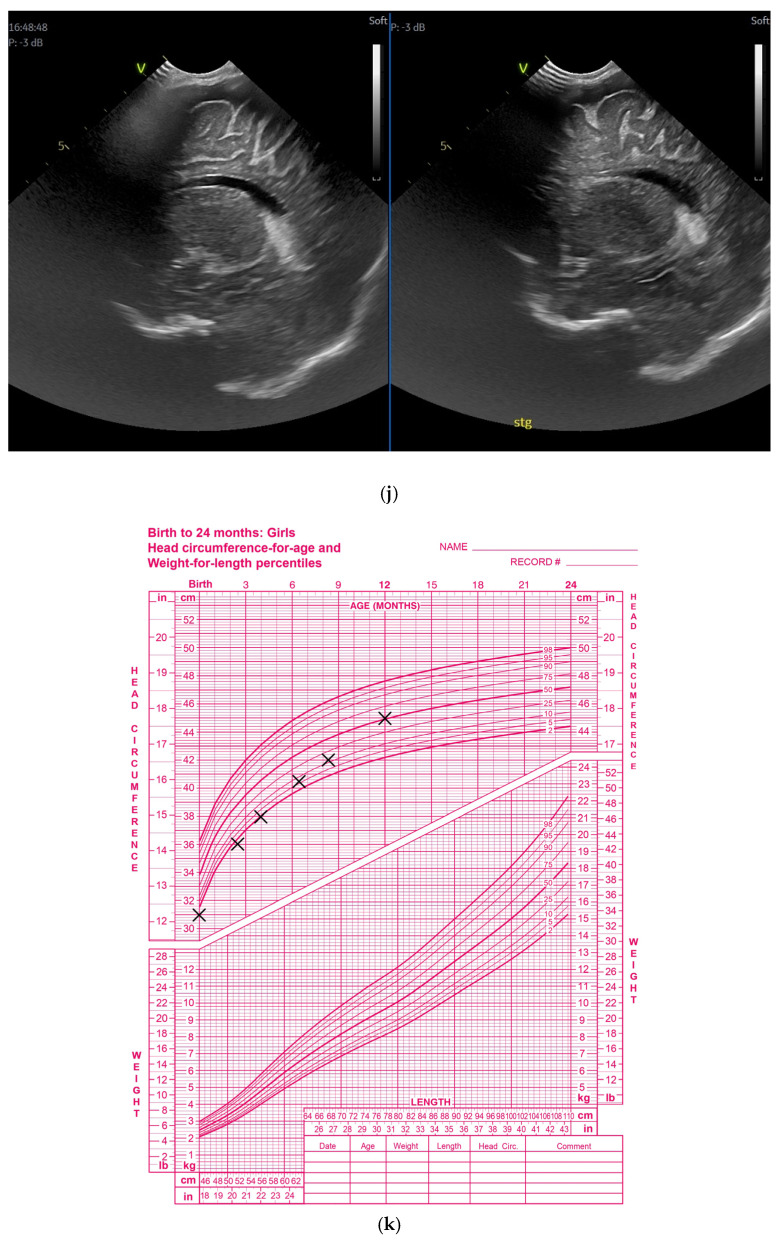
(a) Day one. Cranial ultrasound. Sagittal view. A left frontal conatal cyst can be observed. IHF—interhemispheric fissure. CG—cingulate gyrus. CC—corpus callosum. CSP—cavum septum pellucidum. TCG—thalamic-caudate groove. MF—Monroe foramina. SF—Sylvian fissure. Yellow arrow—conatal cyst (personal image collection). (b) Day three. Cranial ultrasound. Coronal view. Bilateral ventricular dilatation. Yellow arrows—hyperechoic images can be seen at the level of the thalamic-caudate groove and within the ventricles (personal image collection). (c) Day three. Cranial ultrasound. Coronal view. Orange arrows—bilateral periventricular hyperechoic images (personal image collection). (d) Day three. Cranial ultrasound—Doppler examination. Sagittal view. CC—corpus callosum. FL—frontal lobe. LV AH—lateral ventricle anterior horn. V 3—third ventricle. V 4—fourth ventricle. OL—occipital Lobe. Yellow arrow—presence of color Doppler signal within the Sylvian aqueduct (personal image collection). (e) Day 19. Cranial ultrasound. Sagittal view (left). Yellow arrows—conatal cysts. Pink arrows—PVL lesions (personal image collection). (f) Day 19. Cranial ultrasound. Sagittal view (left). Yellow arrows—conatal cysts. Pink arrows—PVL lesions (personal image collection). (g) Follow-up examination at term corrected age. Cranial ultrasound. Coronal view. Slight dilation of the lateral ventricles. IHF—interhemispheric fissure. CG—cingulate gyrus. CC—corpus callosum. CSP—cavum septum pellucidum. LV—lateral ventricle. MF—Monroe foramina (personal image collection). (h) Second follow-up examination. Cranial ultrasound. Coronal view. Dilation of interhemispheric fissure and subarachnoid space. D1—sinus–cortical width measurement. D2—interhemispheric width measurement. D3—cranio-cortical width measurement (personal image collection). (i) Third follow-up examination. Cranial ultrasound. Coronal view (left). D1—dilation of interhemispheric fissure. D2, D3—Levine index measurement (personal image collection). (j) Cranial ultrasound. Sagittal view (right). Normal brain structures (personal image collection). (k) Head circumference-for-age. “X” symbols represent the measurements performed from birth and follow-up examinations (personal image collection) [28].
